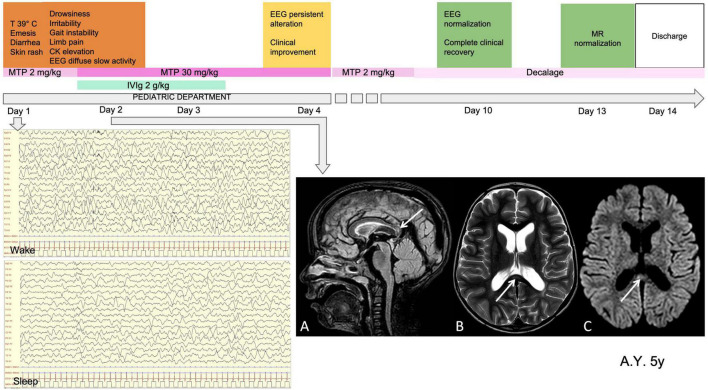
Clinical, MRI and EEG findings in a representative patient with diffuse EEG alterations. Male, 5 years old. MISC presentation characterized by fever (T 39^°^C), gastrointestinal involvement, skin rash, neurological symptoms (drowsiness, irritability, gait instability, limb pain) and CKelevation. Laboratory data at onset WBC 3420 (10^A^3/mmc) (N 1810, L 1470); PLTs 224 (10^A^3/mmc); Hb 11.9 (g/dL); CRP 33 (mg/l); ferritin 829 (mcg/l); D-dimer 12077 (mcg/l); NT-ProBNP 1982 (ng/l); TnT 820 (ng/l). On day 1 he was given IVIg (2g/kg for 2 days) and on day high-dose steroid treatment was started (MTP 30 mg/kg for 3 days). MRI performed on day 2 (A). Sagittal FLAIR image, (B) Axial T2-weighted images show slight focal median hyperintense lesion in the splenium of corpus callosum (arrows). Parenchymal signal abnormality and thickness reduction can be observed in the peritrigonal white matter on the left side in (B). (C) Axial diffusion- weighted image shows reduction of proton diffusivity at the site of the CC lesion (arrow). EEG performed on day 1 (D). Diffuse high-amplitude delta slow activity (E). Loss of organization of sleep activity, with high-amplitude delta slow waves. Clinical and EEG normalization was observed on day 10 and normalization of MRI on day 13. CK, creatine kinase; WBC, white blood cells; N, neutrophils; L, lymphocytes; PLTs, platelets; Hb, hemoglobin; CRP, C-reactive protein; NT-ProBNP, amino-terminal fragment of the brain natriuretic peptide; TnT, troponin T; IVIg, intravenous immunoglobulin; MTP, methylprednisolone; MRI, magnetic resonance imaging; CC, corpus callosum; EEG, electroencephalography; PICU, pediatric intensive care unit admission.
